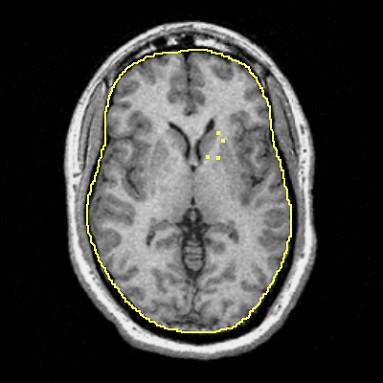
Axial MRI with four corner markers of the internal capsule placed.
